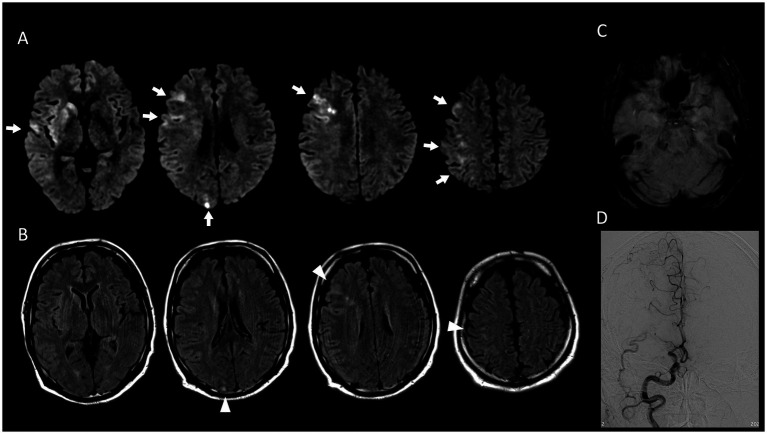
A representative case with high ICAS-M score. DWI (*b* = 1,000 s/mm^2^) revealed multiple cortical/border-zone infarcts in the right middle cerebral territory (white arrows, A). Corresponding FLAIR images of the same slices demonstrate mixed acute-subacute infarcts within the same vascular territory (white arrowheads, B). The DWI-ASPECT score was 5, indicating infarction in the caudate, lentiform, M2, M3, and M5 regions. Susceptibility-weighted imaging (gradient-echo sequence, slice thickness 5 mm) shows no susceptibility vessel sign in the right M1 segment (C). Digital subtraction angiography confirms occlusion of the right middle cerebral artery (D). DWI, diffusion-weighted imaging; FLAIR, fluid-attenuated inversion recovery; ASPECTS, Alberta Stroke Program Early CT score.
